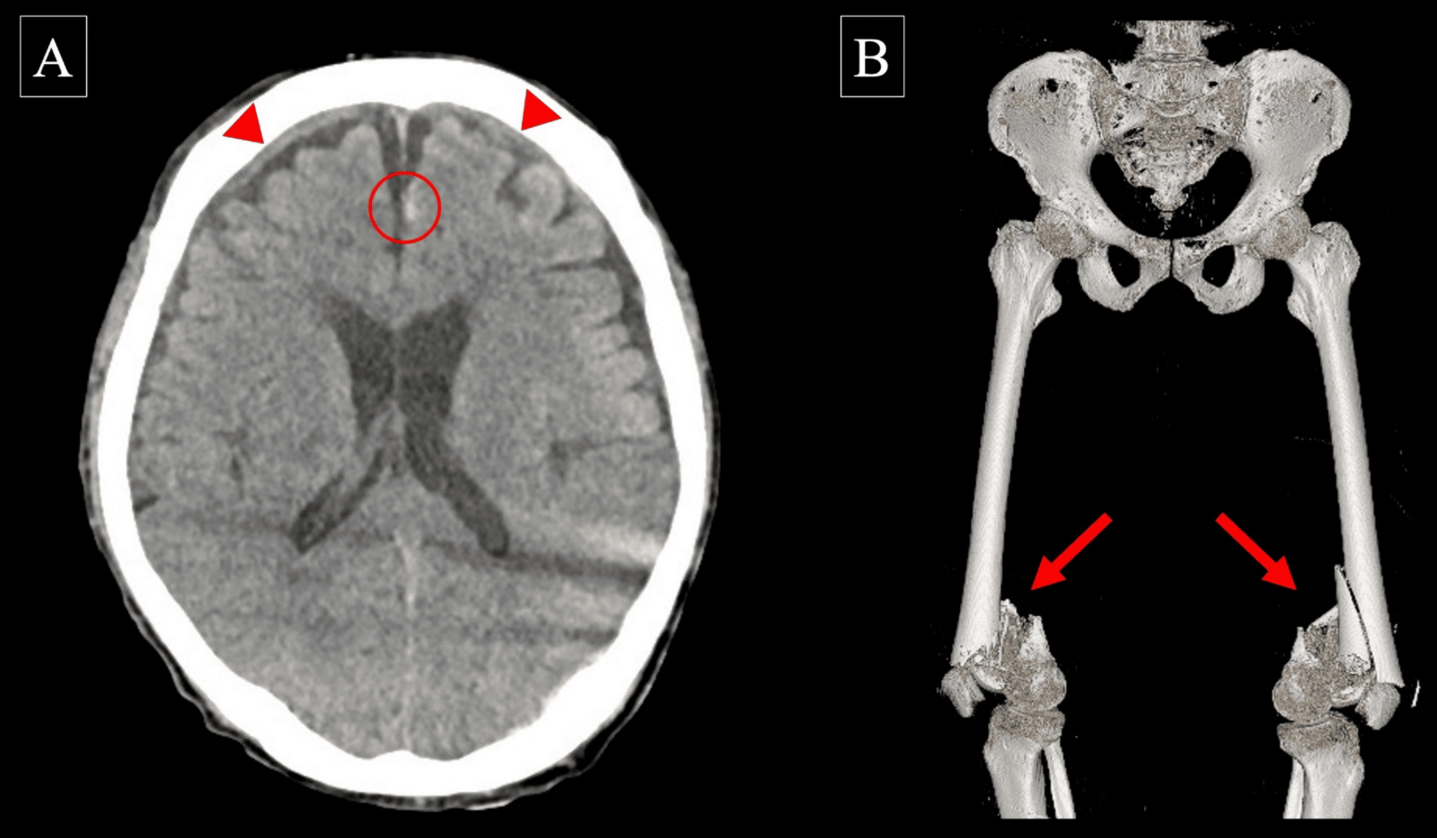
Head (A) and femur (B) CT images prior to the start of ECMO in case 1. The CT shows a traumatic subarachnoid hemorrhage (circle), acute subdural hematoma (arrowheads), and bilateral distal femur fractures (arrows). ECMO, extracorporeal membrane oxygenation; CT, computed tomography
